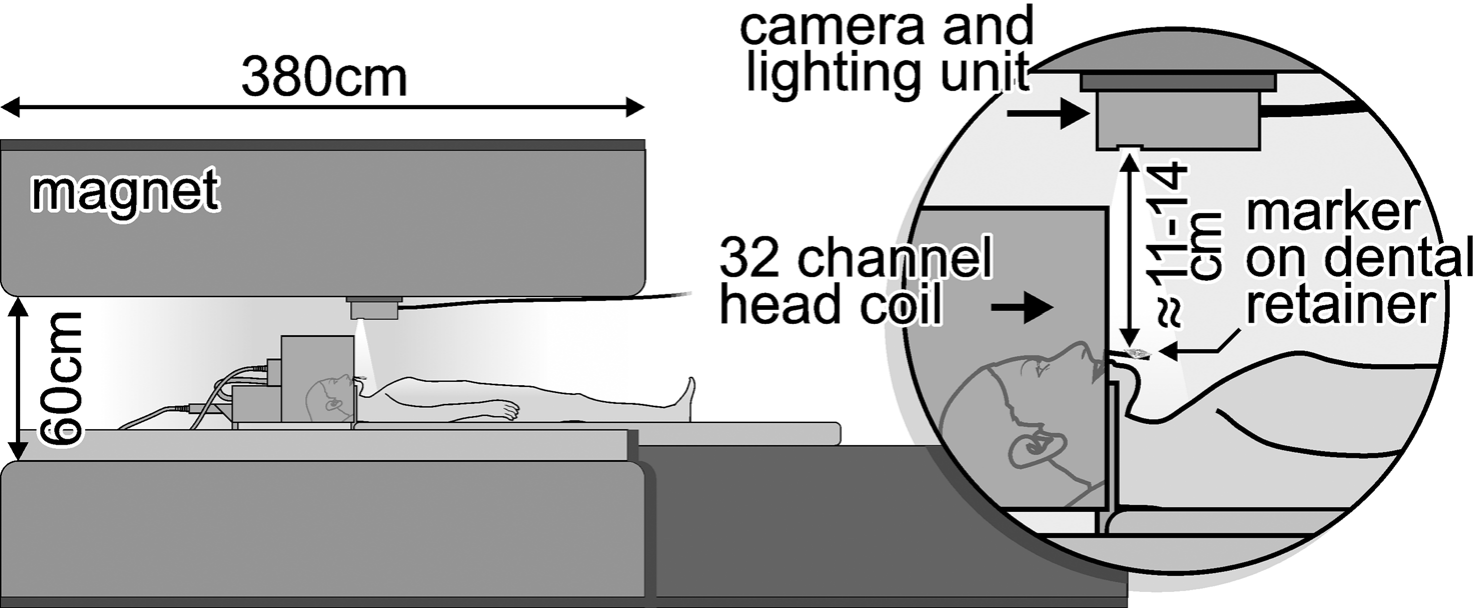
Experimental setup for motion tracking during the MRI measurements. The tracking systems CLU was mounted on the inner cover inside the magnet bore. The MPT marker was presented at a distance of ≈ 11–14 cm from the camera on the extension of the dental brace reaching out of the 32-channel helmet-design head coil.
